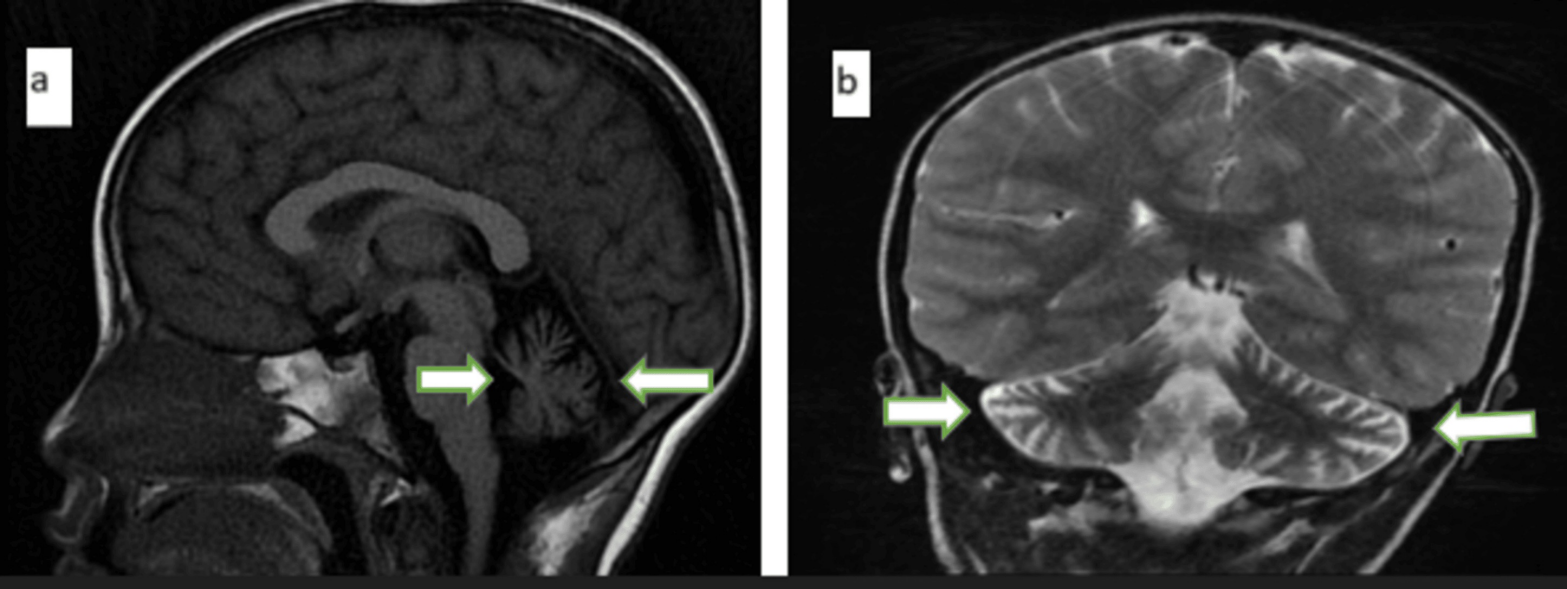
MRI of the brain (a) MRI of the brain (T1): sagittal image of the brain showing pronounced atrophy of the cerebellum. (b) MRI of the brain (T1): axial image of the brain showing pronounced atrophy of the cerebellum, most marked in the vermis.
